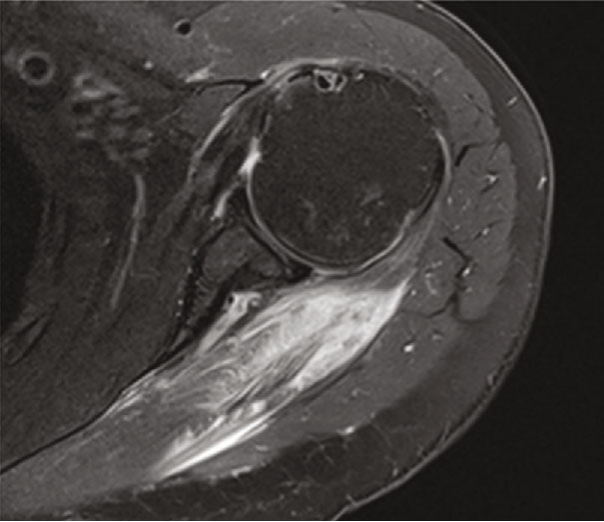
Axial T2 MRI of the left shoulder demonstrating hyperintensity throughout the infraspinatus muscle, consistent with myositis.
